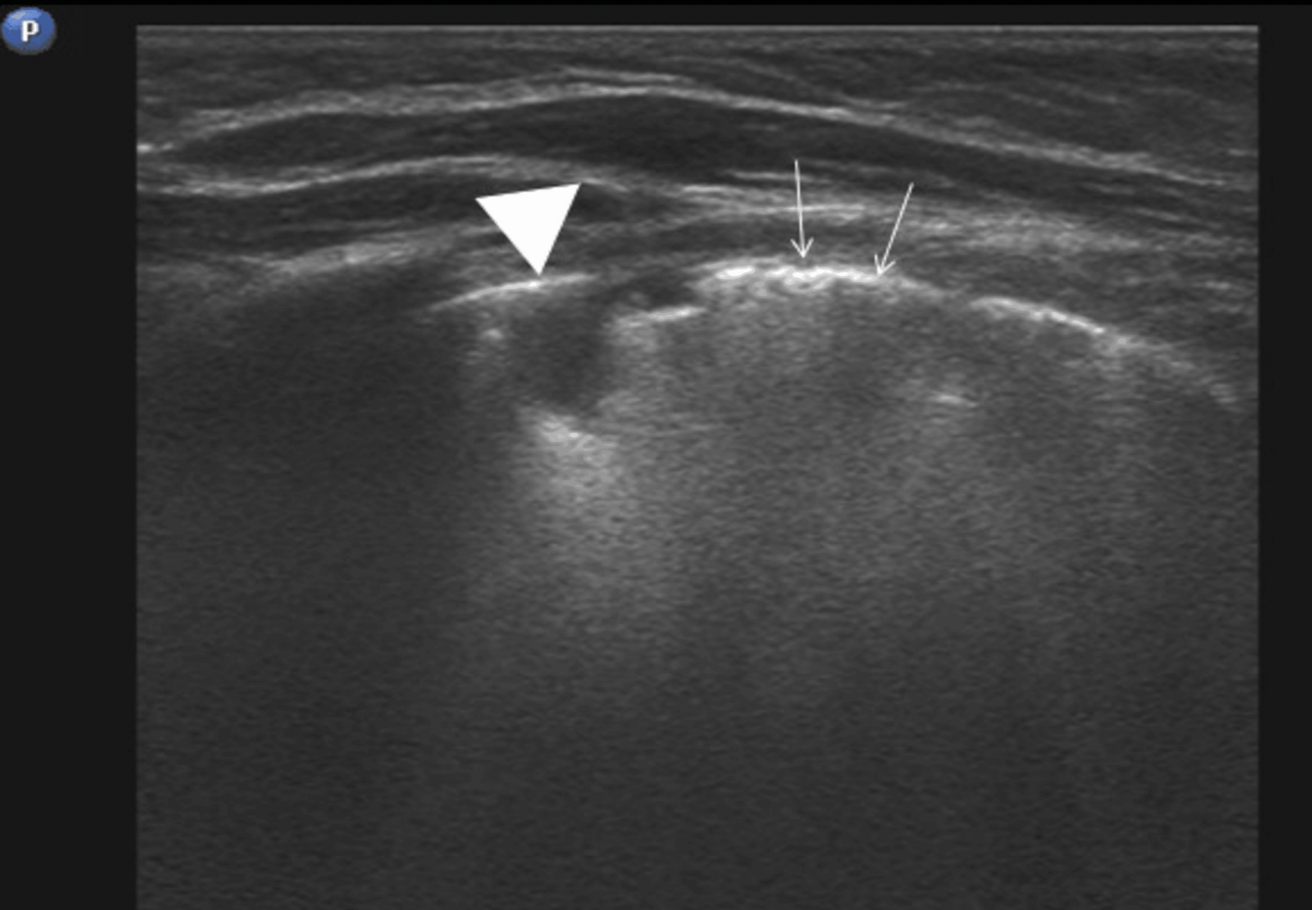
Follow-up LUS (one month) Follow-up lung ultrasound (LUS) reveals residual consolidation (arrowhead: “shred sign”) and coalescent B-Lines (arrows). The bronchogenic cyst is not visualised due its deeper location.
